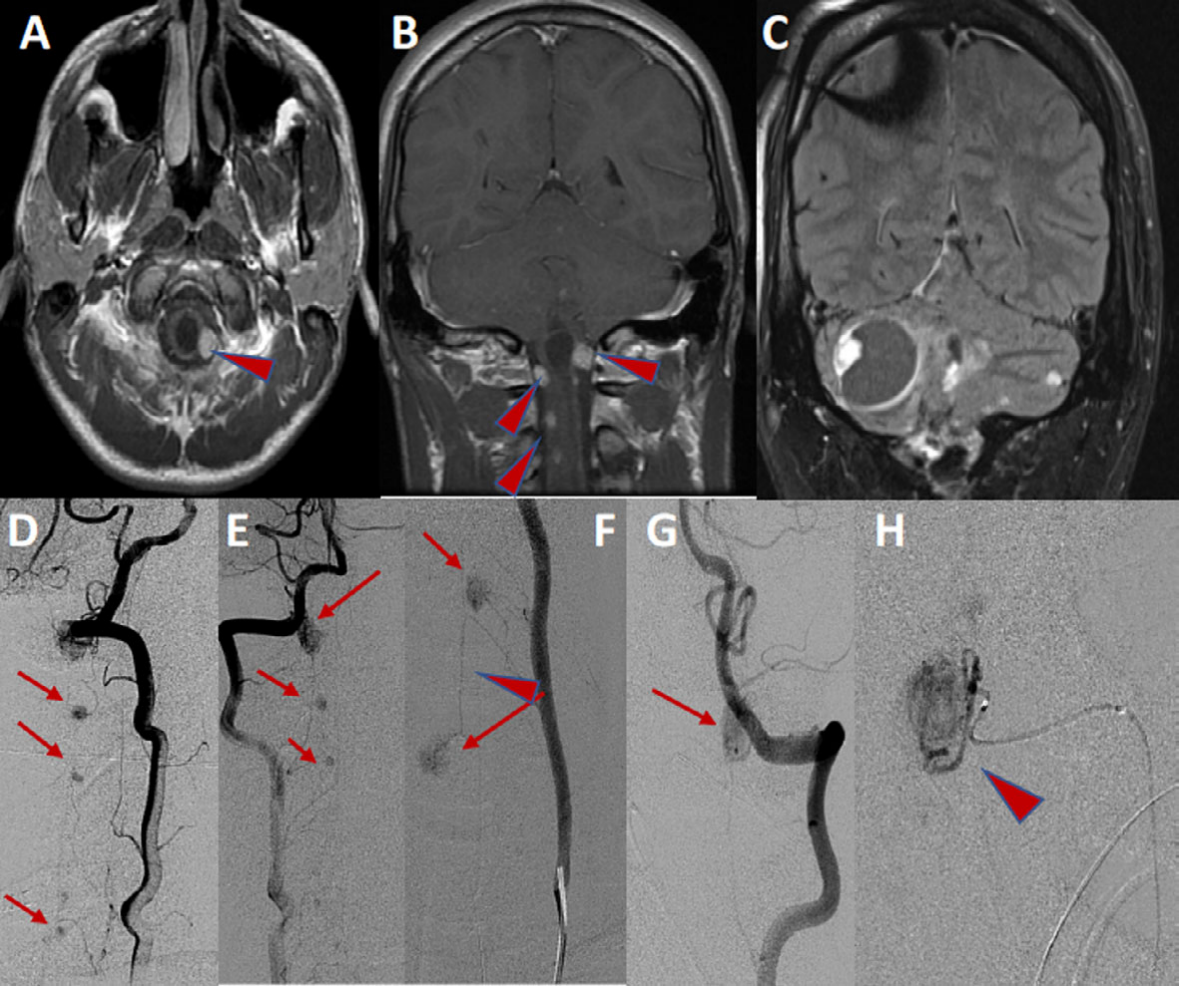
MRI axial and coronal postcontrast T1‐weighted images demonstrating multiple enhancing intra‐axial cerebellar nodules and nodules on the pial surface of the spinal cord (arrowheads) (A, B). MRI coronal postcontrast T1W image demonstrating classic “cyst & mural nodule appearance” of right cerebellar lesion (C). Vertebral artery DSA demonstrating multiple hypervascular nodules (arrows) along the cervical cord consistent with hemangioblastomas (D, E). Left vertebral artery injection with multiple hemangioblastomas, with ASA (arrowhead) supply (F, G). Figure H shows microcatheter injections of segmental artery supply to larger hemangioblastoma at the Craniocervical junction.
